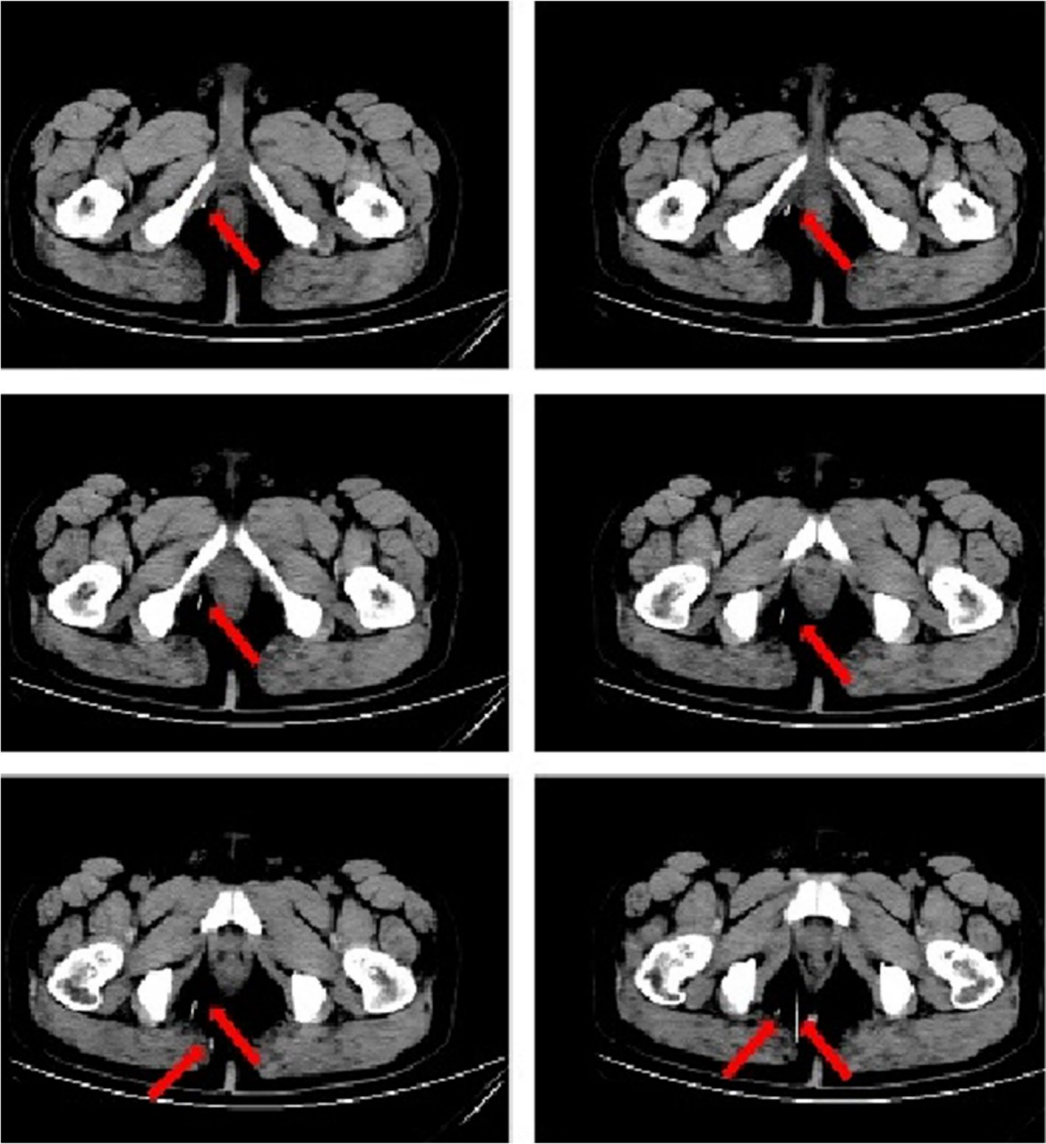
July 31, 2023, CT of the pelvis. The arrows point to the needles
